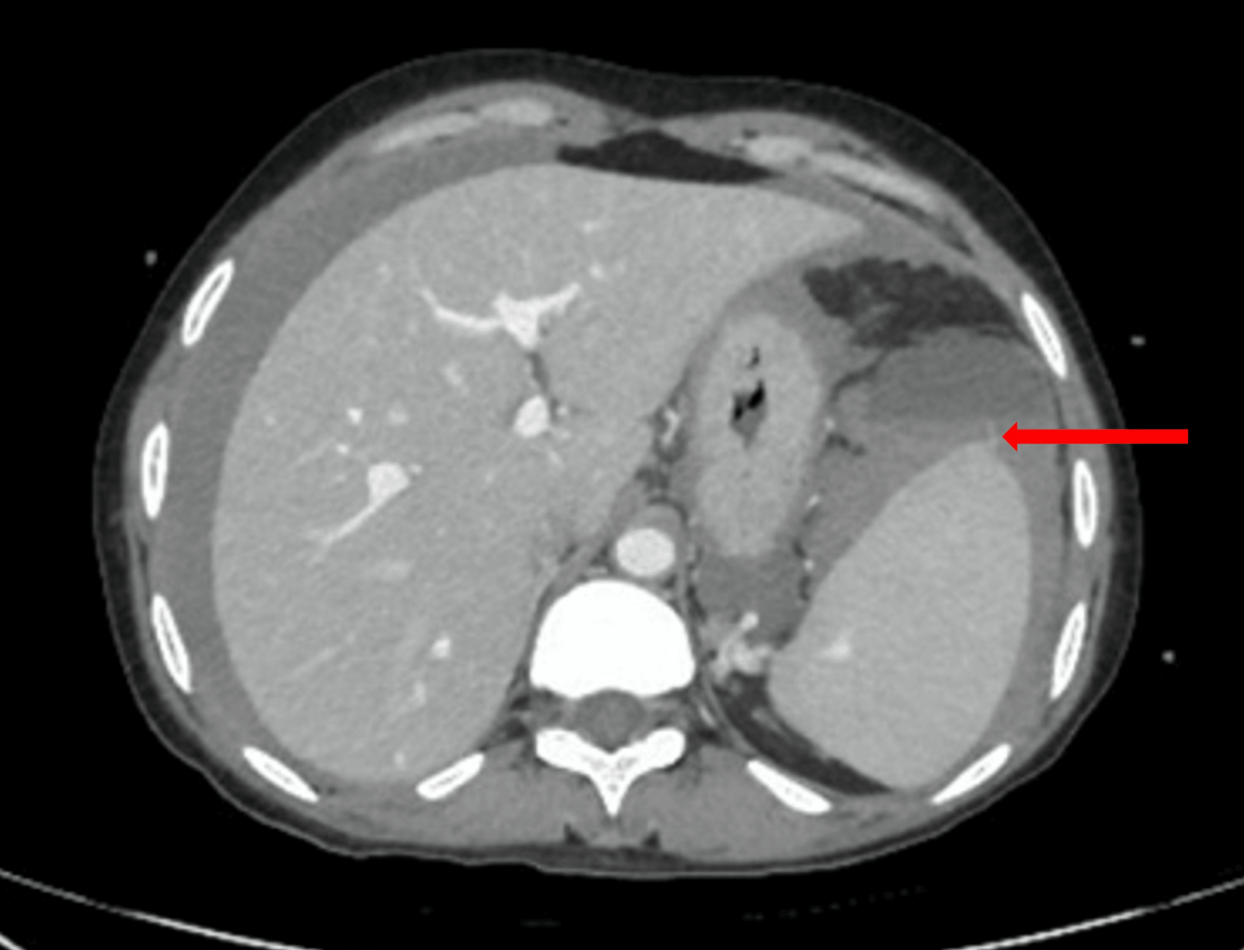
Extravasation seen on CT (red arrow) with hemoperitoneum Axial image of an abdominal CT scan showing active leakage (extravasation) from the spleen, with hemoperitoneum.
